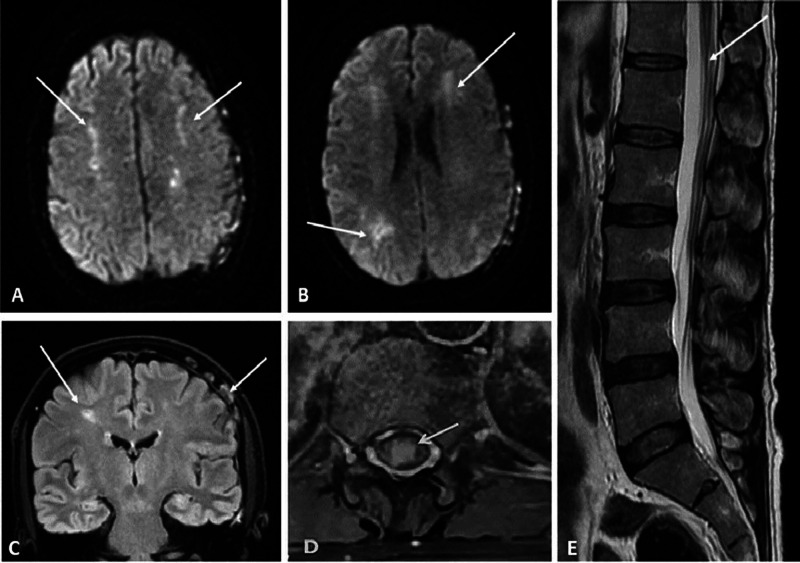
Magnetic resonance imaging of the brain and lumbosacral spine. (A, B) Diffusion-weighted imaging, multiple bilateral asymmetrical predominantly subcortical hyperintense white matter lesions. (C) FLAIR multiple and bilateral hyperintense lesions in the subcortical white matter of the bilateral centrum semiovale and periventricular areas with infiltration of the internal table of the skull. (D) Axial and (E) sagittal T2 weighted imaging signal change in the conus medullaris which enhanced after gadolinium administration.
